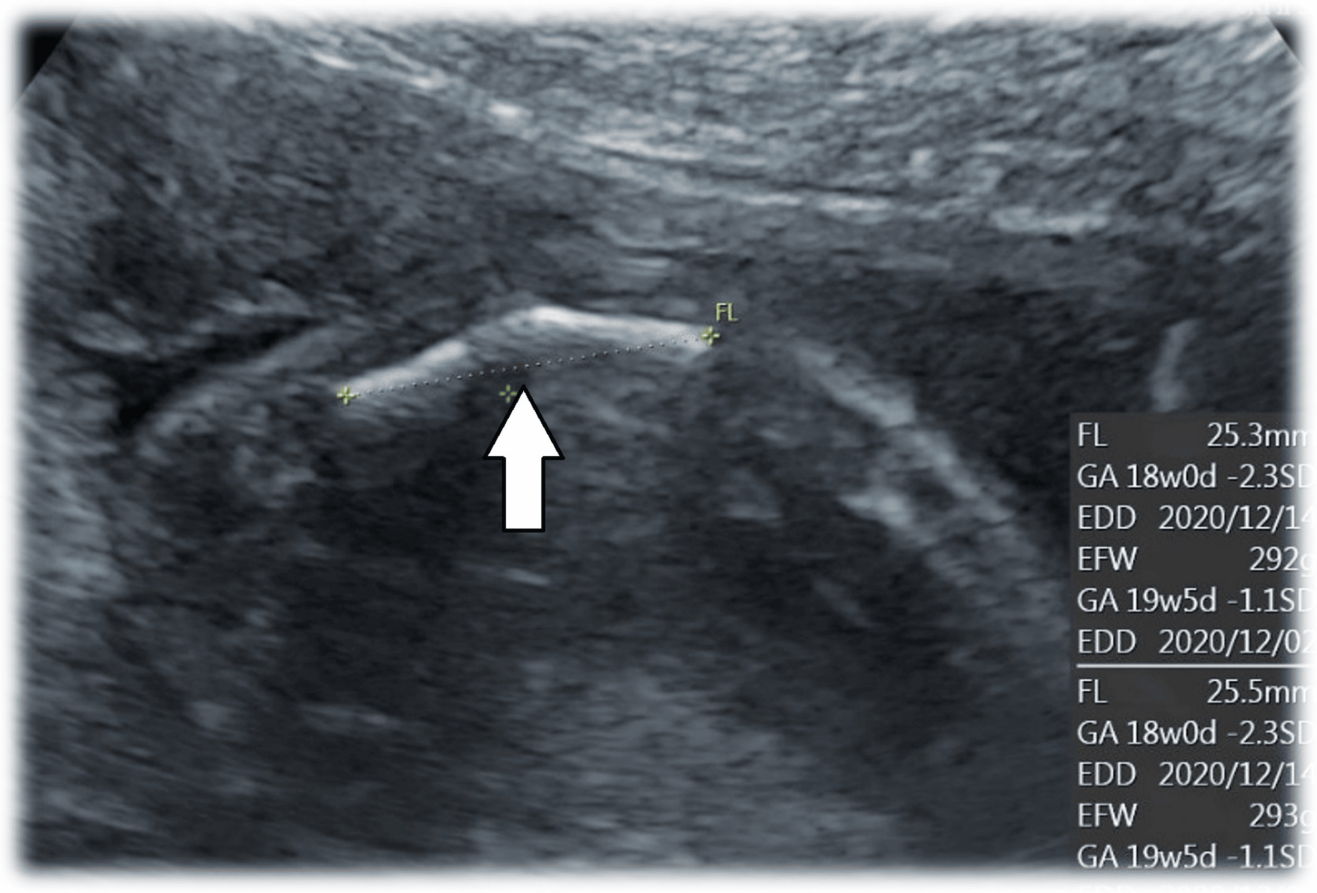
Femur length on ultrasonography of the second pregnancy at 20 weeks and four days of gestation The fetal femur was shortened and curved (white arrow).
